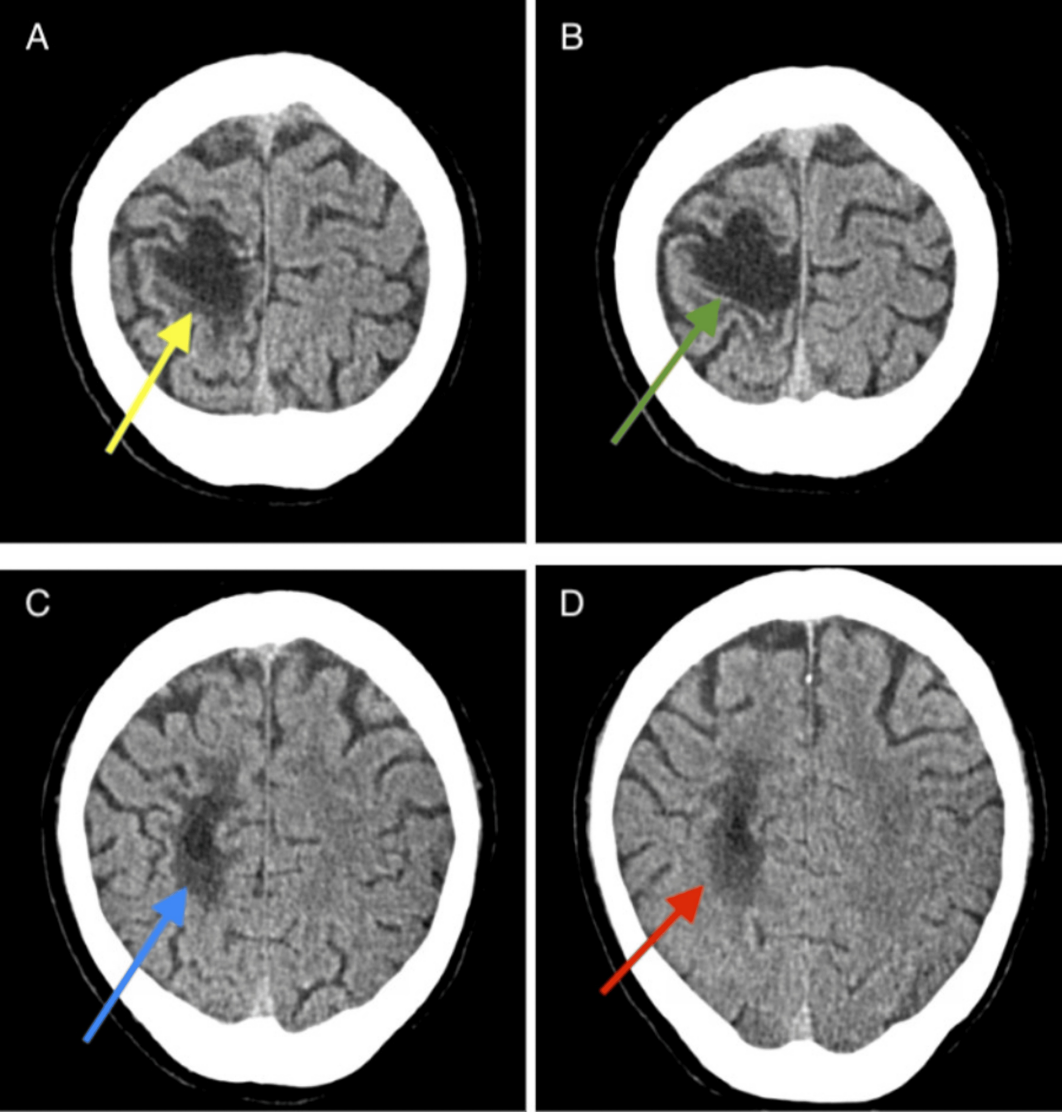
CT head without contrast Non-contrast axial CT scan of the head demonstrating a deformity in the calvarium just above the right frontal convexity region denoted by yellow and green arrows (A, B), suggesting previous trauma. Additionally, encephalomalacia in the right frontal convexity region is denoted by blue and red arrows (C, D), characterized by an area of low attenuation consistent with loss of brain tissue and gliosis. No acute hemorrhage or mass effect is present.
